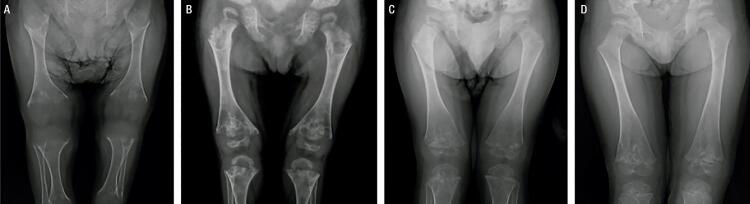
Lower limb radiographs. (A) Age 1 year and 2 months, prior to treatment. (B) Age 2 years and 10 months, just prior to treatment with asfotase alfa. (C) Age 4 years, after 1 year of treatment. (D) Age 5 years, after 2 years of treatment. Improvements similar to those in the upper limbs also occurred in the lower limbs.
